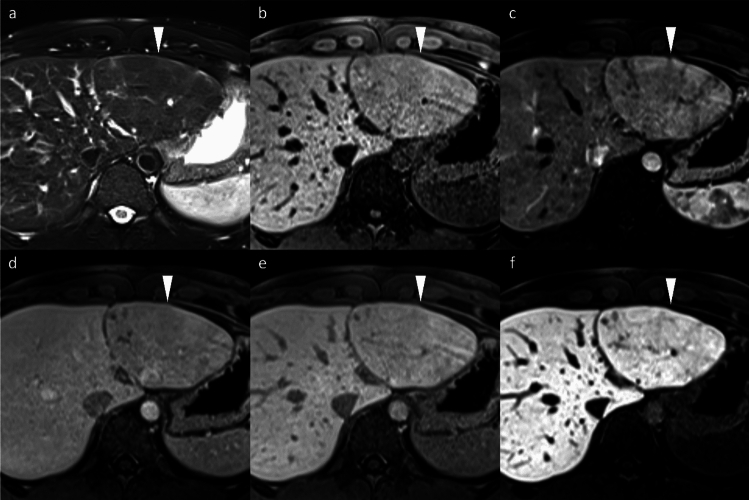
β-Catenin-mutated hepatocellular adenoma in a 23-year-old man. A mass (arrowheads) located in the lateral segment demonstrates an isointense signal on both the fat-suppressed T2-weighted image (a) and the pre-contrast image (b). The mass displays non-rim arterial phase hyperenhancement (c) without portal venous washout (d) and hypointensity on the transitional phase image (e). The mass shows homogeneous hyperintensity on the hepatobiliary phase image (f). The nodule was pathologically proven to be β-catenin-mutated hepatocellular adenoma following surgical resection. Imaging characteristics do not reliably differentiate this lesion from hepatocellular carcinoma; however, the absence of portal venous washout may serve as a useful distinguishing feature
